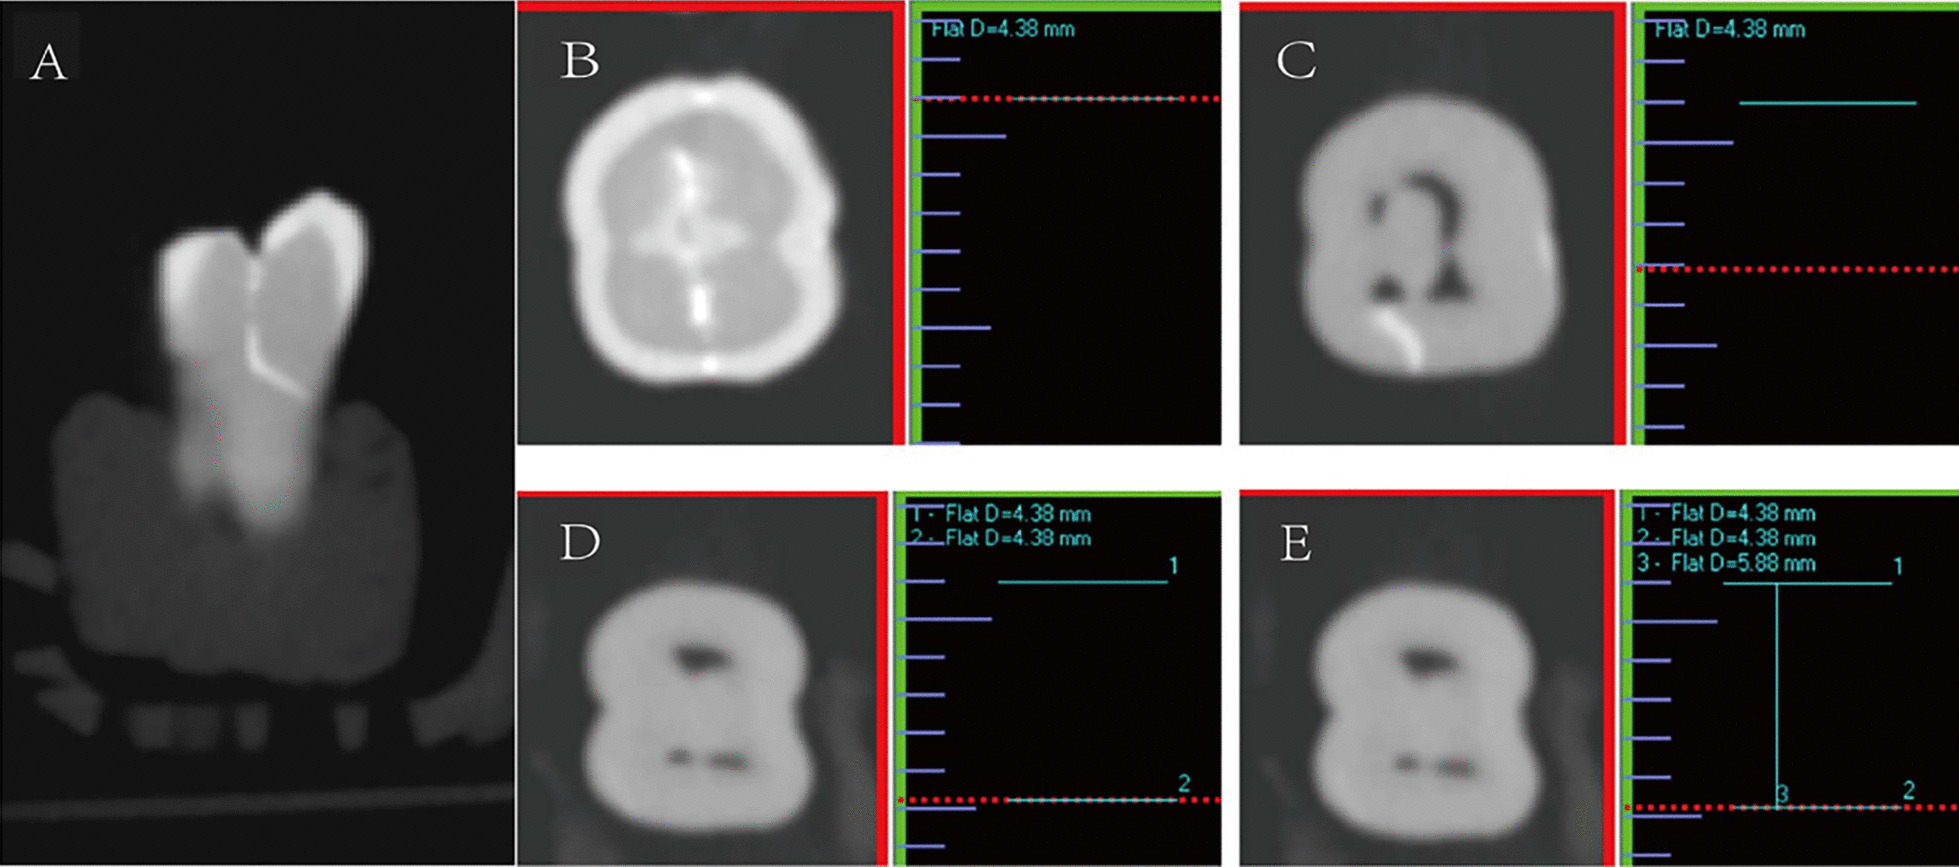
Method of measuring the crack depth of tooth no. 23 on contrast-enhanced cone beam computed tomography (contrast-enhanced CBCT). A The coronal plane of the crack shows the path of the crack. B The first location where the high-density crack started to appear on the contrast-enhanced CBCT scan is marked “1” in the horizontal plane. C A high-density crack image of a cracked tooth. D The crack completely disappeared and is marked “2”. E The distance from “1” to “2”,i.e., the length of segment “3”, was taken as the extension depth of the crack
